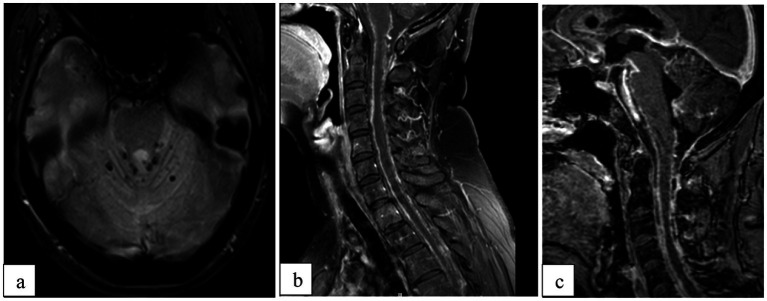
(a) Baseline T2-W GRE MRI at the level of the posterior fossa demonstrates multiple hypo-intense foci in the cerebellar hemispheres consistent with micro-bleeds. (b) Baseline T1-W sagittal MRI of the spinal cord with gadolinium contrast demonstrates diffuse leptomeningeal enhancement involving the cervical spinal cord and brain, with a hyper-intense lesion in the central cord at C4–C7 consistent with myelopathy. (c) Follow-up T1-W TFE sagittal MRI with gadolinium contrast after 5 years demonstrates slight increase of the cervical cord myelopathic lesion (now extending to C7), with persistent leptomeningeal enhancement from the foramen magnum to Th4. MRI, magnetic resonance imaging; T2-W GRE, T2-weighted gradient echo; T1 W, T1-weighted; TFE, turbo field echo; C4–C7, Th4, cervical (C4–C7) and thoracic (Th4) vertebral levels.
